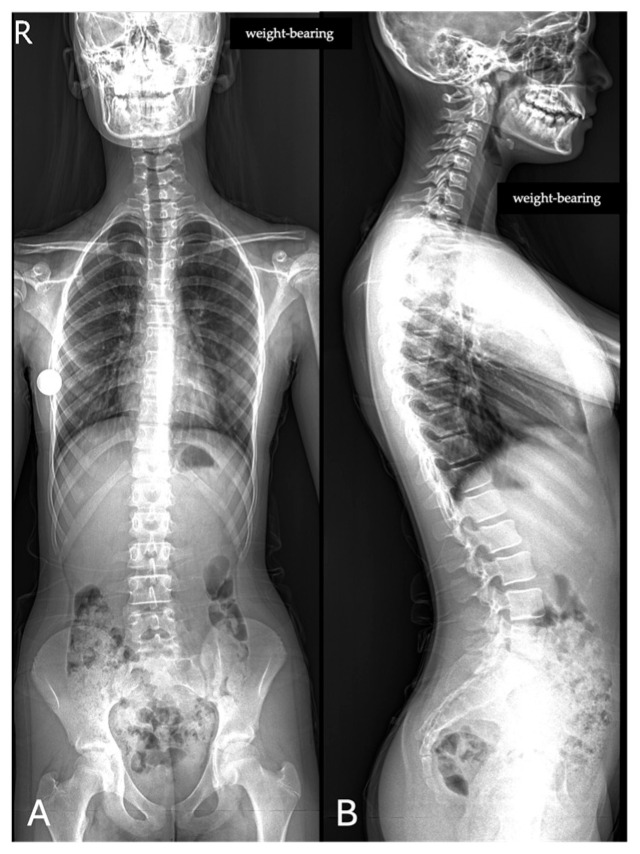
Full-spine radiographs were acquired in standing position using the same dedicated scanner (Bloomix 120 ED-R). (A) Anteroposterior (AP) view; (B) lateral view. For each patient, AP and lateral projections were obtained from the base of the skull to the last sacral segment, including the hip bones and femoral heads. The scanner enabled the acquisition of the entire spine in a single shot, without the need for image stitching.
